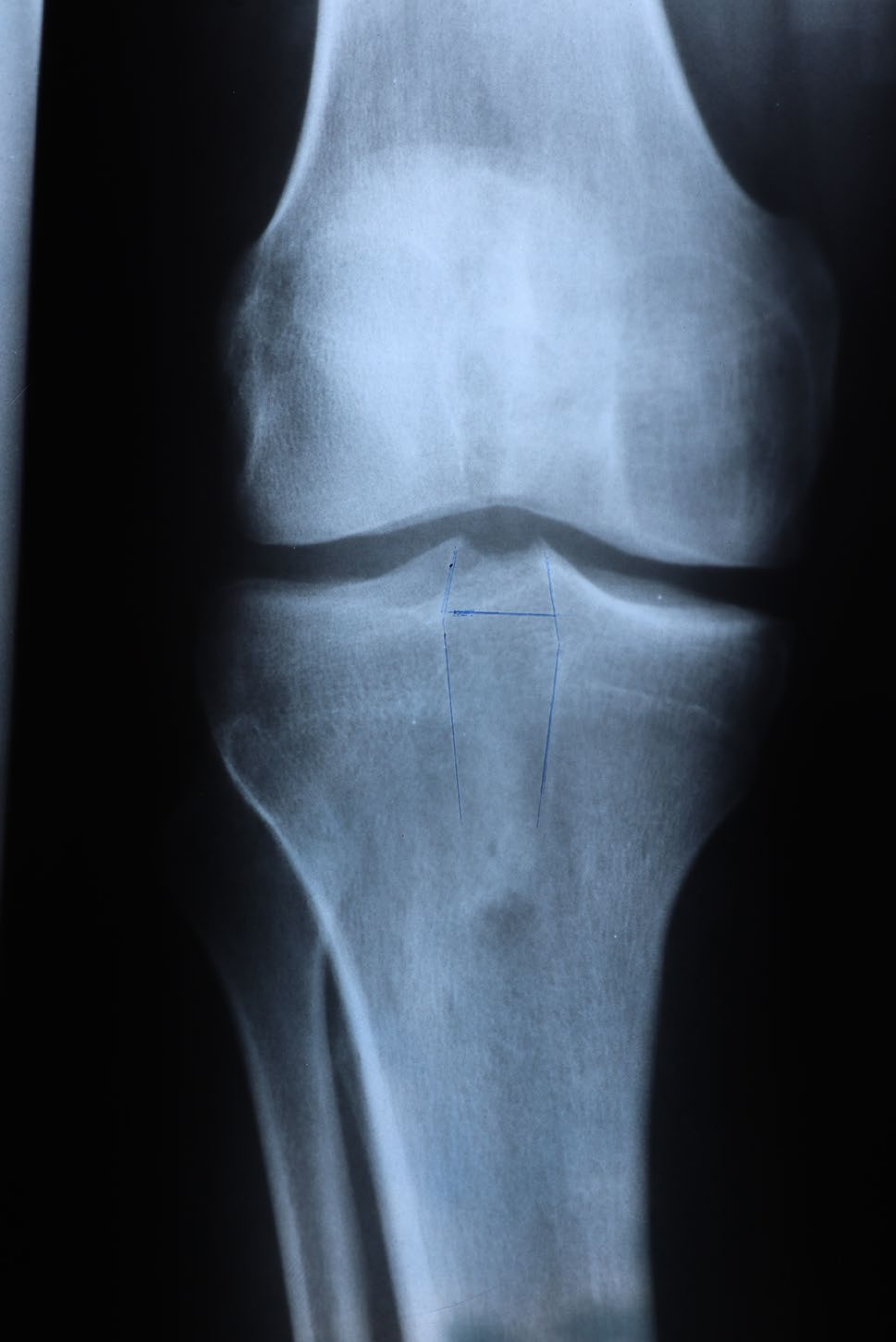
Tunnel widening on the AP X-ray, using PT graft
